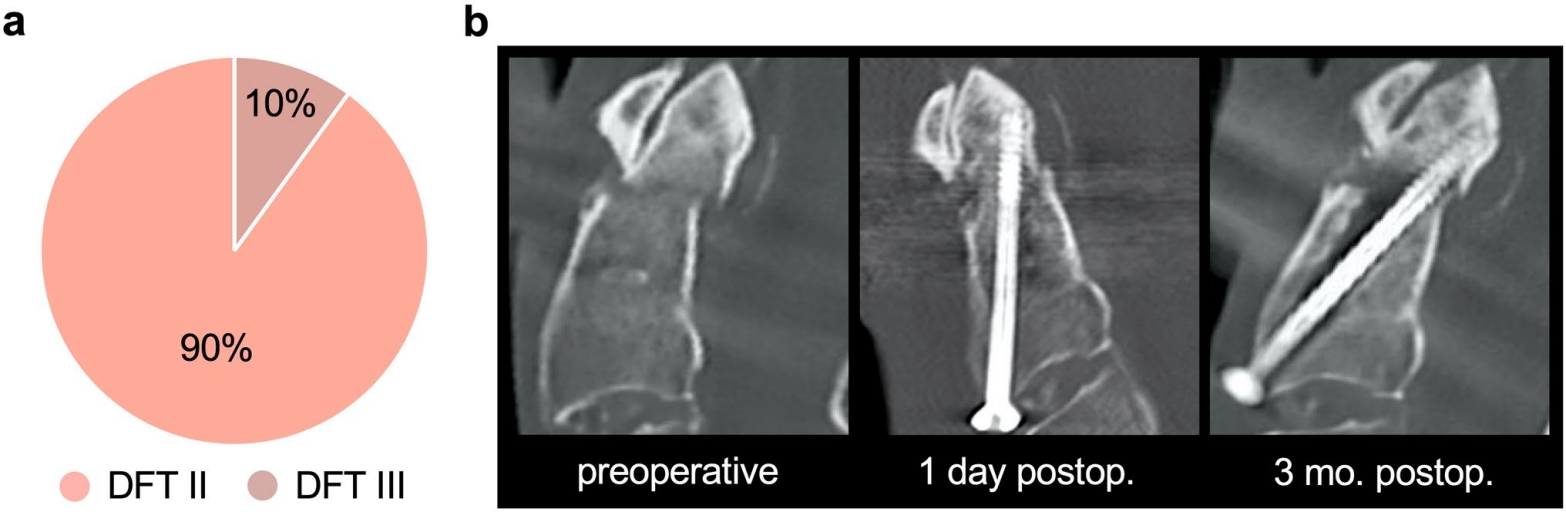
Clinical characteristics of axis fractures. a Distribution of fracture types in patients treated surgically at our institution (*n* = 78). DFT II-Anderson and D'Alonzo fracture type II, DFT III–Anderson and D’Alonzo fracture type III. b Left panel: Preoperative sagittal reformats of CT images showing a dens axis fracture type II according to Anderson and D’Alonzo (DFTII). Middle panel: Postoperative result after osteosynthesis using a Böhler screw. Right panel: Follow up after 3 months postoperatively with insufficient bone healing and pseudarthrosis accompanied by screw loosening
